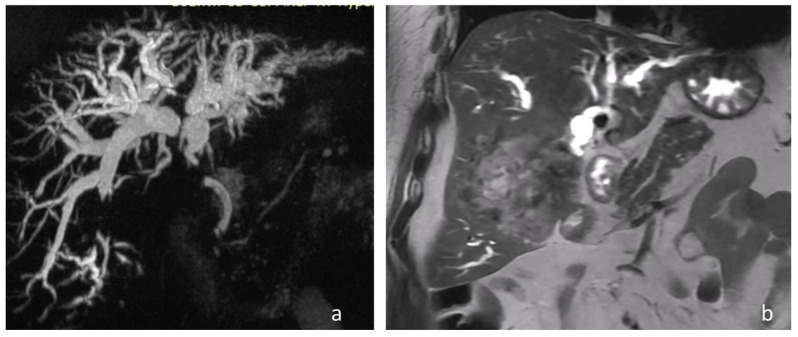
(a) MRCP image showing a hilar biliary obstruction with intrahepatic duct dilatation. (b) MRI image of a HASTE-Coronal sequence shows a large right liver lobe tumor reaching the liver hilum.
